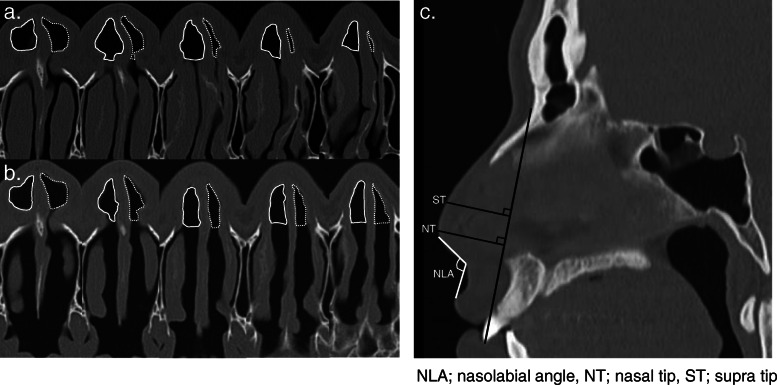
Preoperative (a) and postoperative (b) areas anterior to the anterior edge of the conchal crest were measured by computed tomography. The dotted lines indicate the area of the convex side, and the solid lines indicate the area of the concave side. c For the evaluation of the nasal tip projection, a vertical line was drawn from the line connecting the nasion and maxillary central incisor to the nasal tip and supratip. The distance and nasolabial angle were measured. NLA: nasolabial angle; NT: nasal tip; ST: supra tip
